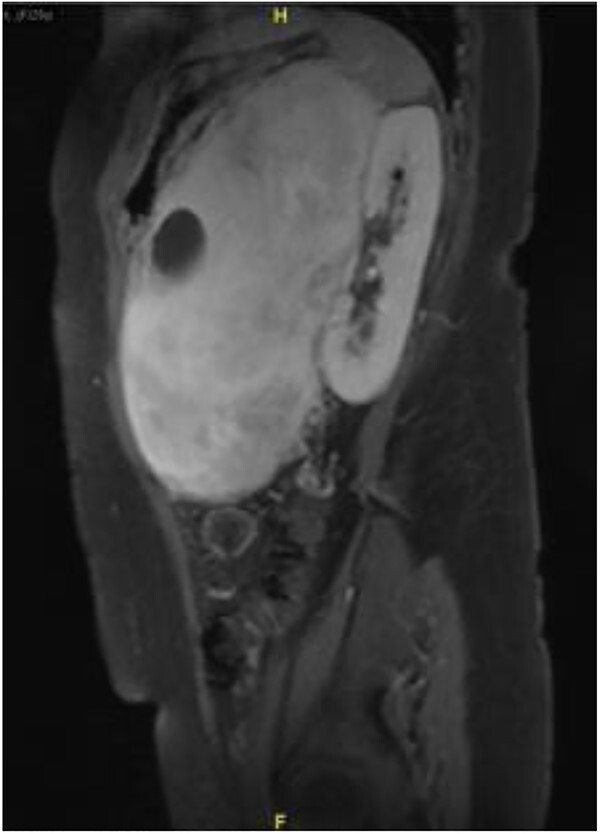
Sagittal view on magnetic resonance imaging abdomen
